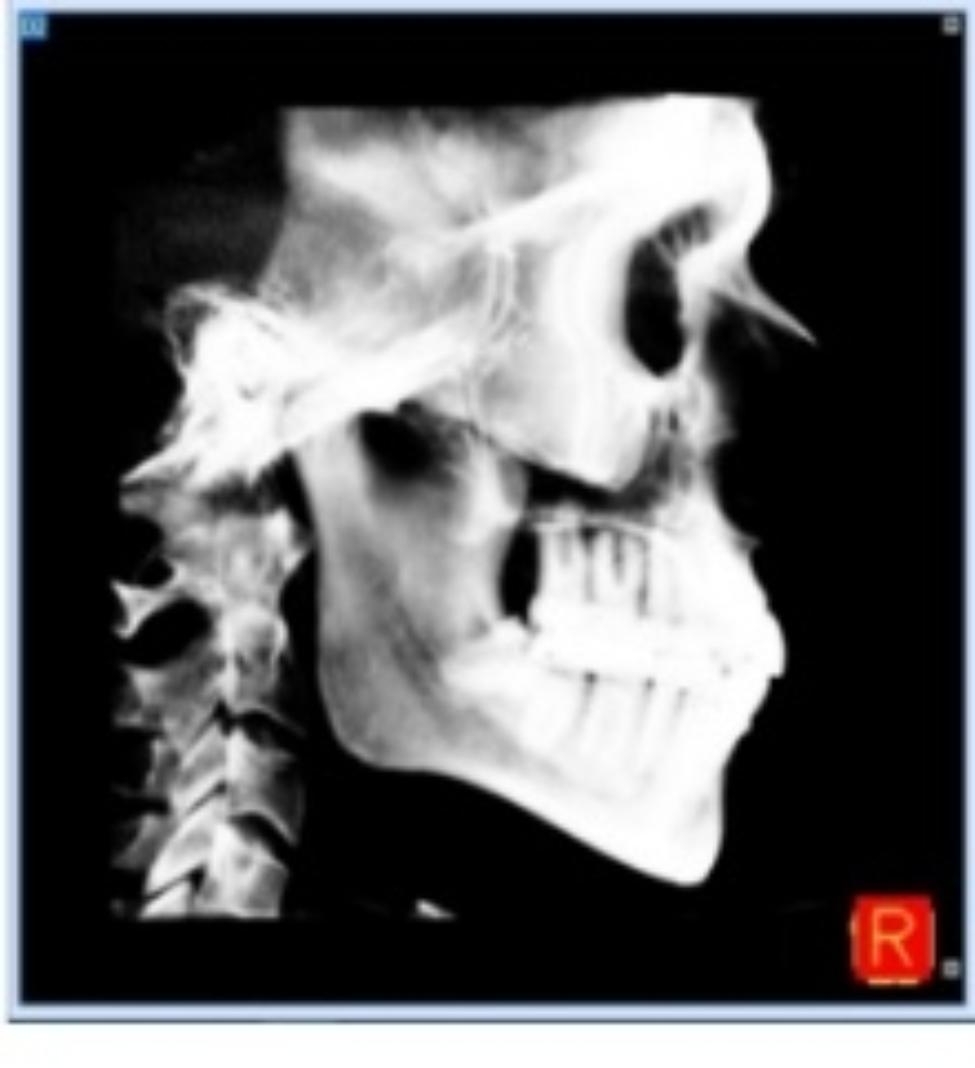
A CBCT synthesized lateral cephalograms
